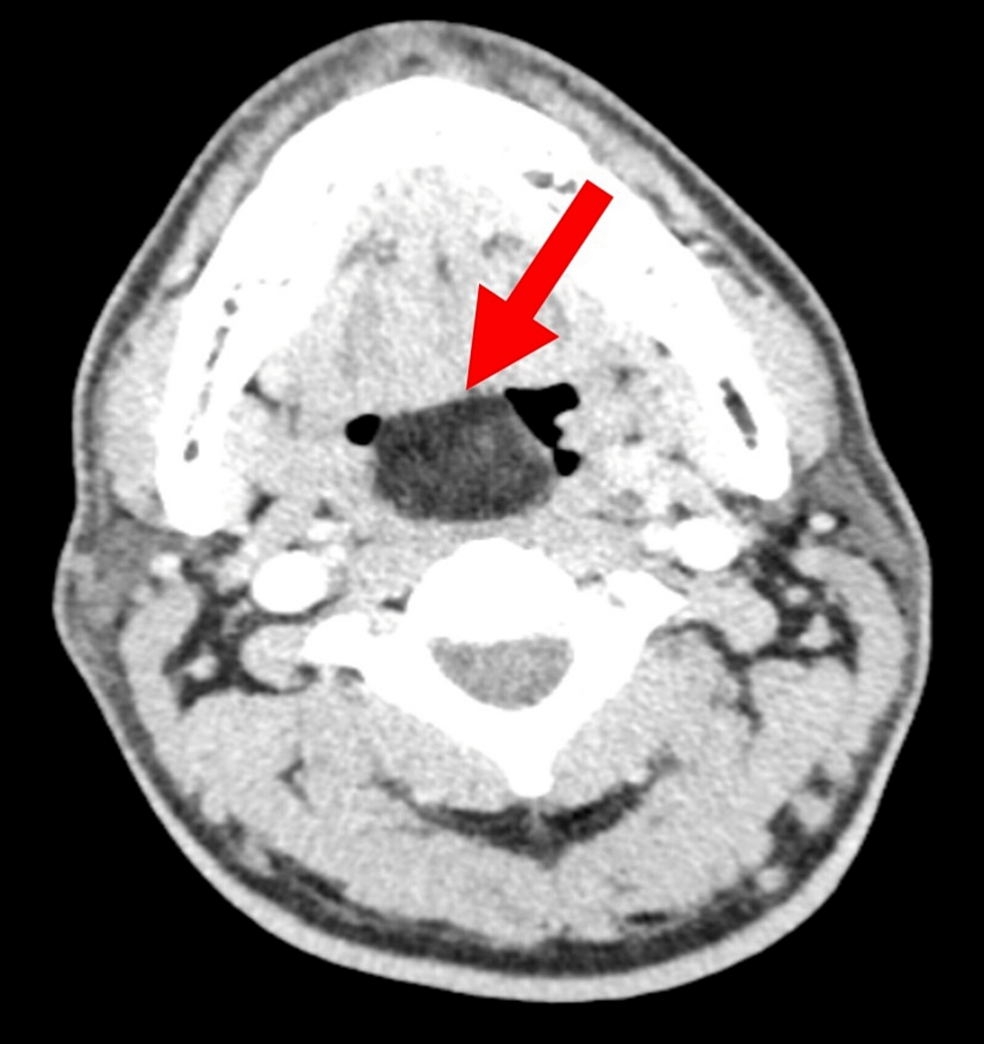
Axial CECT neck showed that the lesion measured approximately 2.6 x 2.6 x 3.0 cm. It had a mean attenuation of -72 to -61HU (suggestive of fat component). CECT: Contrast-enhanced computed tomography.
